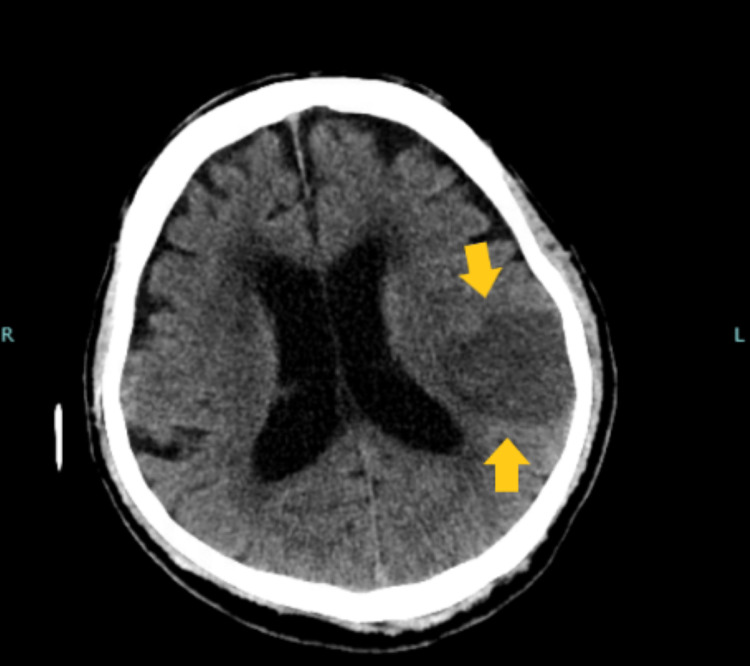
CT scan on the second day of admission showing large evolving infarct within left temporal and left parietal lobe (yellow arrows).
